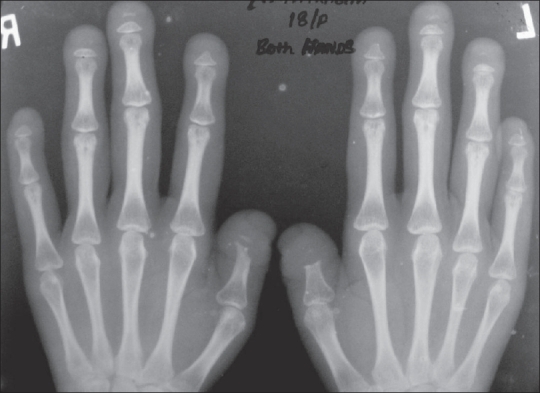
A frontal radiograph of both hands shows acro-osteolysis
